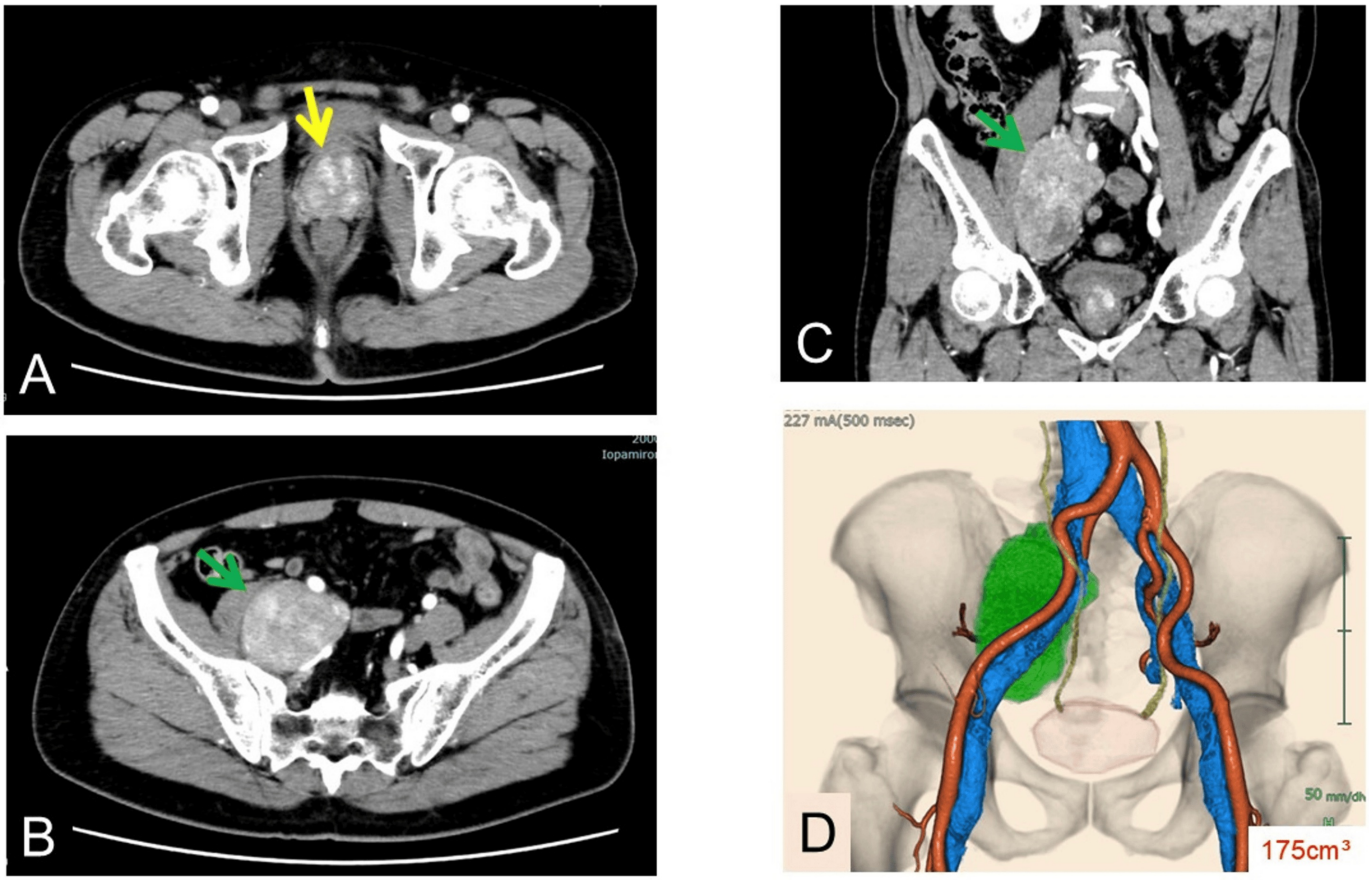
CT images A: Axial contrast-enhanced CT showing the prostate (yellow arrow), measuring approximately 43 × 40 × 39 mm. MRI revealed extracapsular extension on the right lobe, consistent with clinical stage T3a disease. B and C: Axial and coronal CT views showing a right pelvic lymph node metastasis measuring 100 × 62 × 50 mm (green arrows indicate the lymph node). D: Three-dimensional reconstruction using SYNAPSE VINCENT software showing the lymph node volume (green), calculated to be 175 cm³. CT: computed tomography, MRI: magnetic resonance imaging
